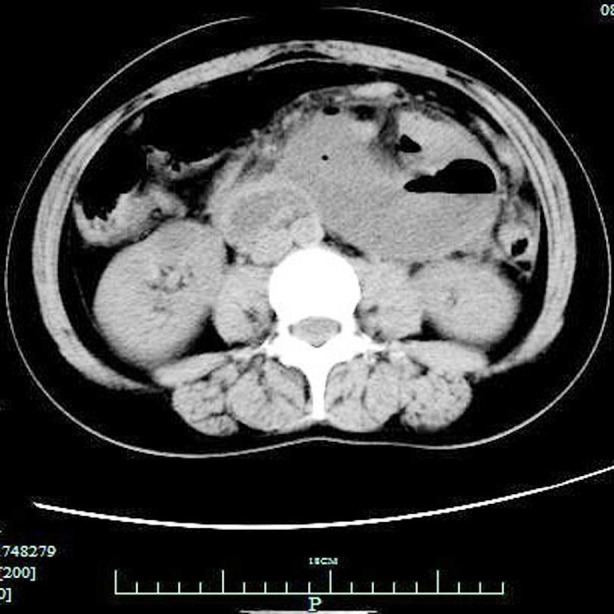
Axial CT image of abdomen demonstrates dilated small bowel loops in the left upper abdominal quadrant combined with air-fluid level.
